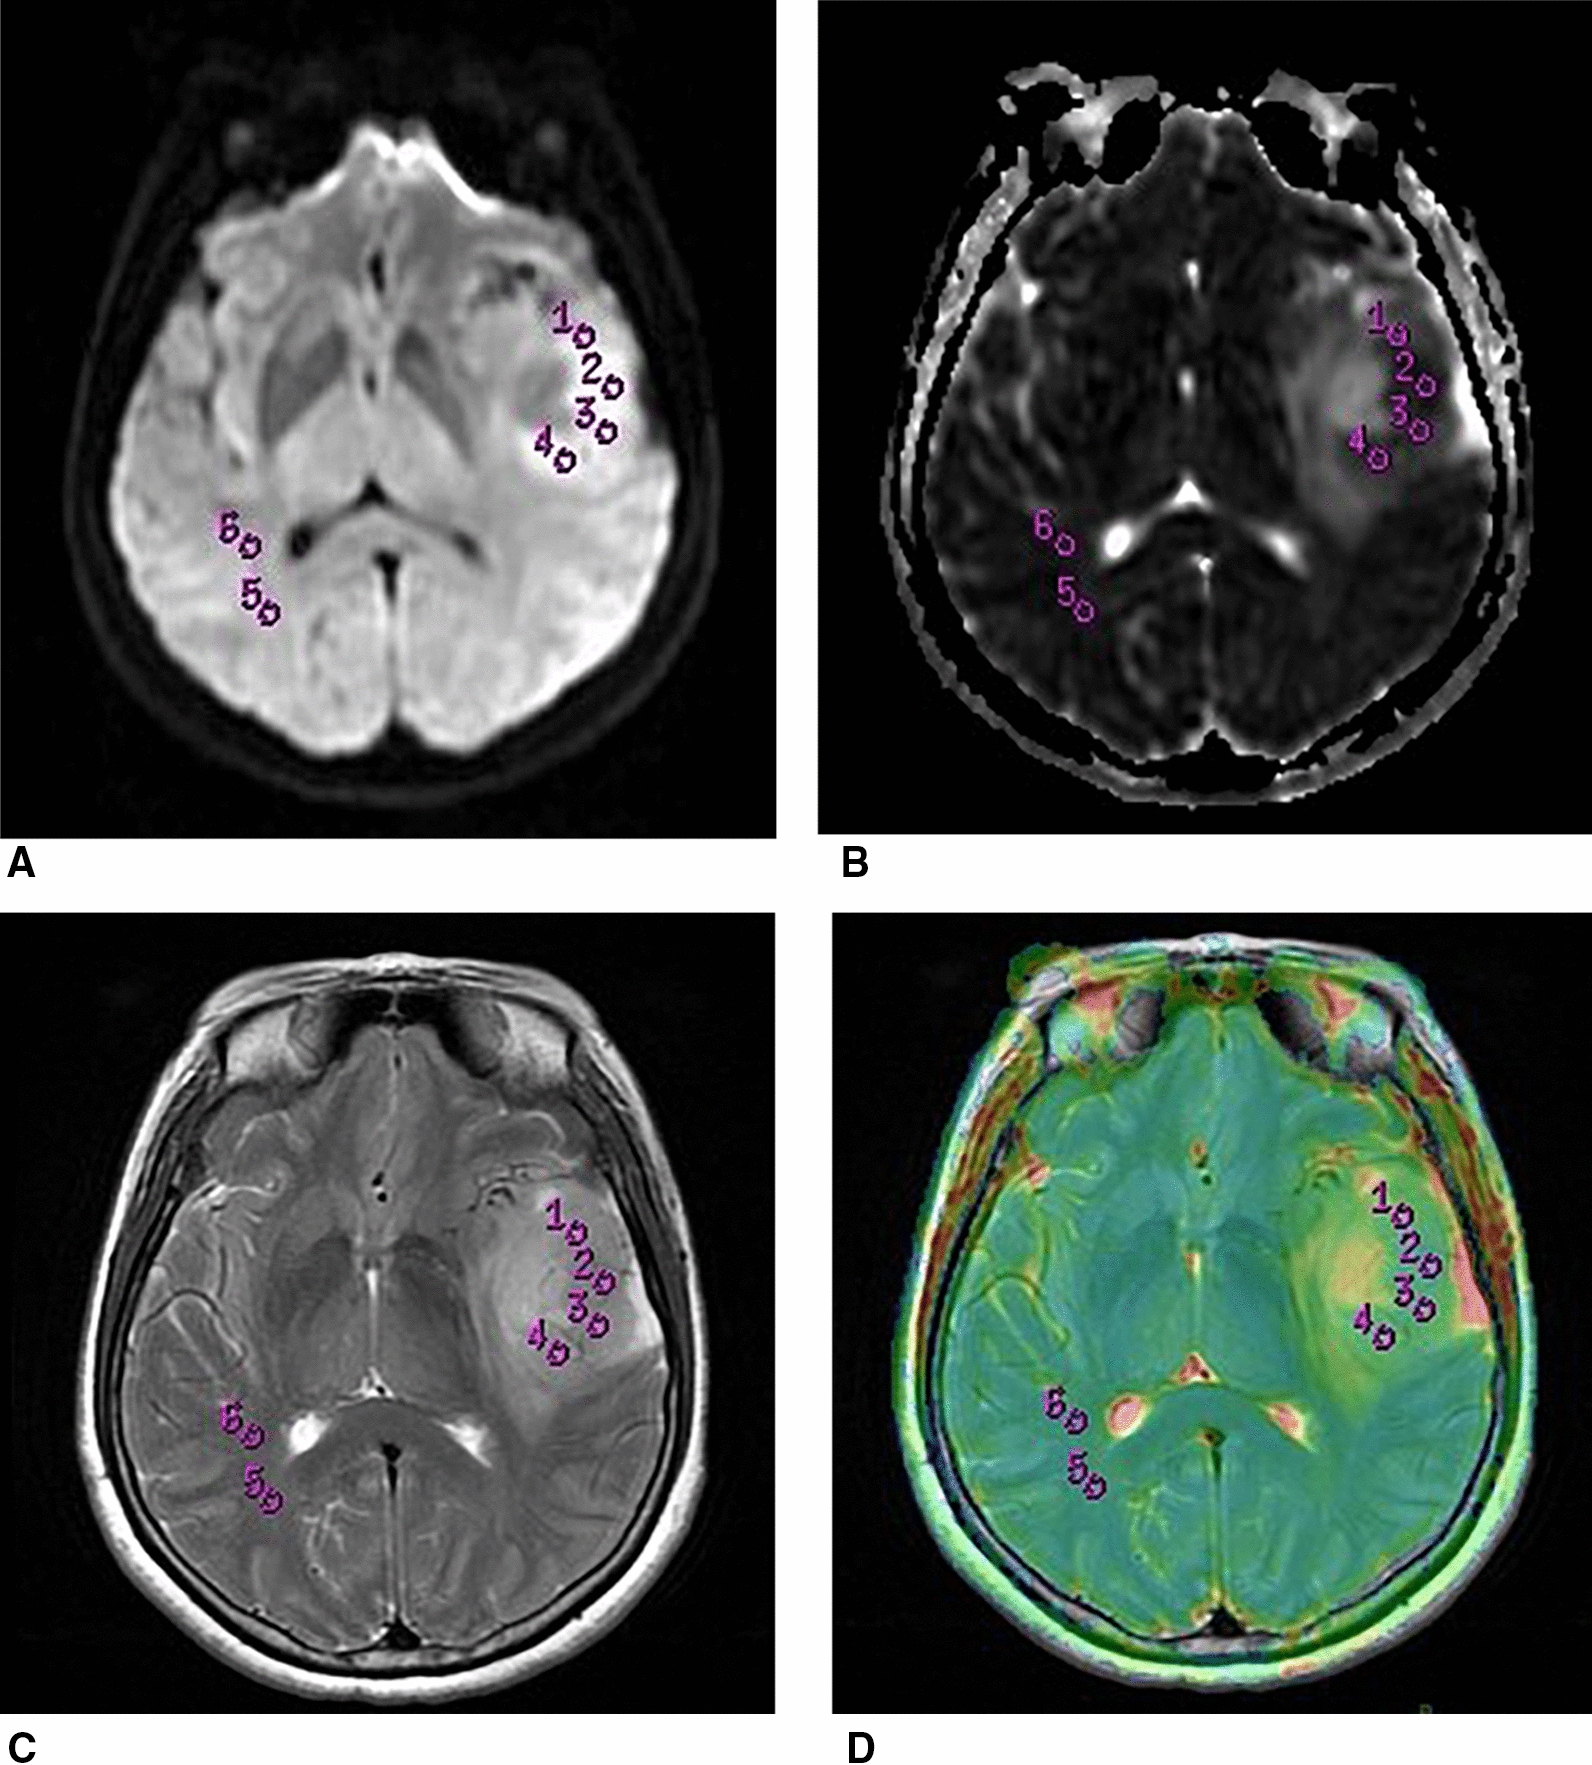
Images showed an example of the placement of ROIs. Axial DWI (A), ADC (B), T2WI (C), and co-registered T2WI (D) from a patient with low grade glioma. Four non-overlapping round ROIs were placed within the solid part of the glioma on co-registered T2WI (ROI 1–4), while two same-sized ROIs (ROI 5–6) were placed within the contralateral white matter to calculate the value of the mean ADC in each ROI. DWI: diffusion-weighted imaging; *ADC* apparent diffusion coefficient; *ROI* region of interest
